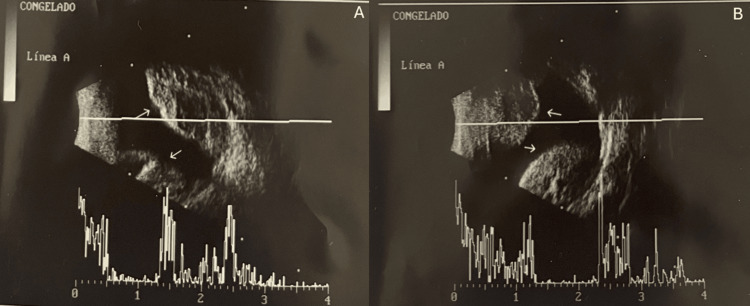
B-scan ultrasonography of the left eye showing a dome-shaped, hyperechogenic elevation (white arrows) in the posterior segment, consistent with suprachoroidal hemorrhage. The presence of opposing choroidal elevations (white arrows) suggests the characteristic “kissing choroidals.”
